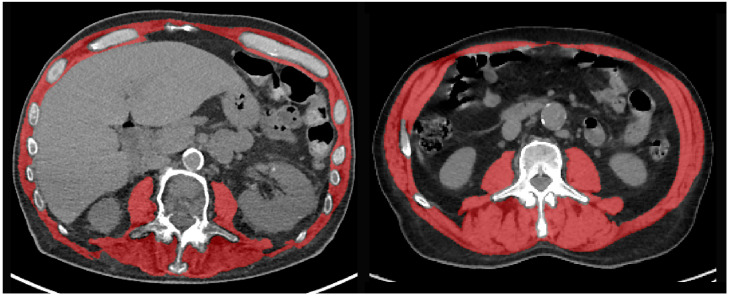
Representative examples of CT images at the L3 vertebral level with segmentation of the skeletal muscle area (red overlay) in two patients with (left) preoperative sarcopenia and postoperative complications, and (right) absence of sarcopenia and favorable outcome after VATS anatomical pulmonary resection.
